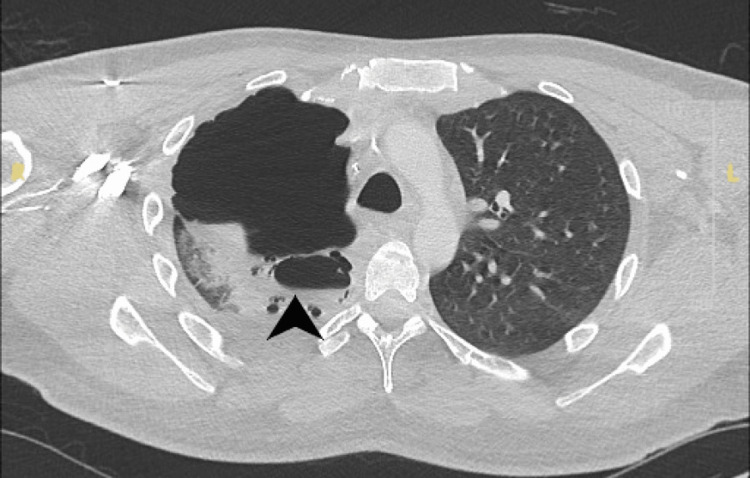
Axial CT thorax demonstrating a complex right-sided multiloculated hydropneumothorax with multiple internal septations. A visible communication between the hydropneumothorax and a cavitating lesion in the right lower lobe (arrowhead) is noted, suggestive of a bronchopleural fistula. CT: computed tomography
